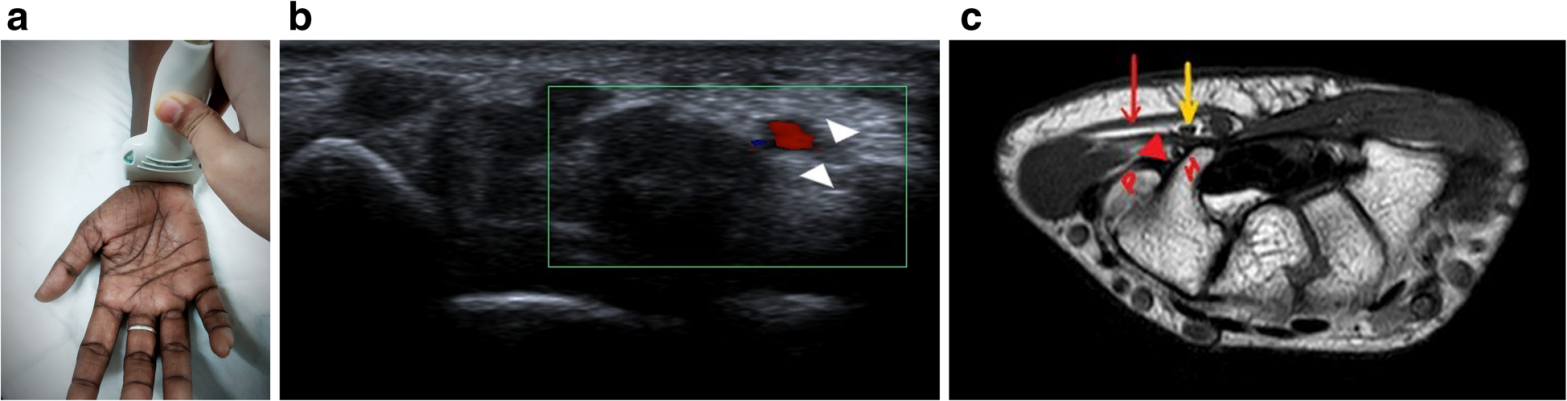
a Probe position for localisation of the ulnar nerve at the wrist. b The nerve (arrowhead) is localised in the short axis in the Guyon’s canal between the pisiform and hook of hamate, lying adjacent to the ulnar artery (colour Doppler signal). c MR image showing the normal ulnar nerve (yellow arrow) in the Guyon’s canal: pisiform (P) and the hook of hamate (H) form the radial and ulnar boundaries. The roof is formed by the volar carpal ligament (red arrow) while the hypothenar muscles and transverse carpal ligament (red arrowhead) form the floor
